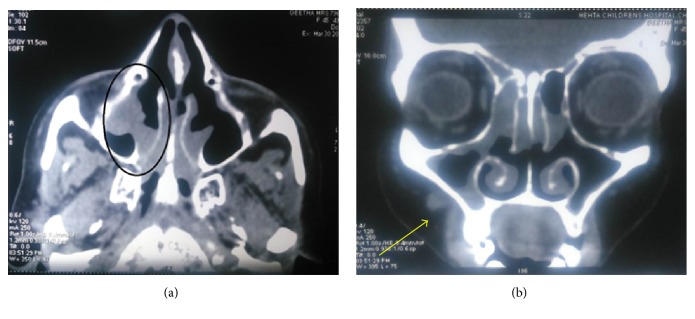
(a) CT image of the lesion: right posterior maxillary region showing irregular soft tissue growth. (b) Coronal CT view in which the location of the haemangioma is being shown by an arrow.
